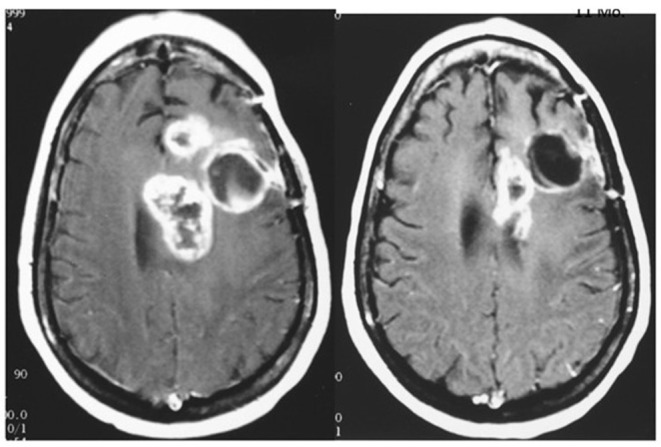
MRI of brain of a 42 year old female with recurrent glioblastoma showing significant mass reduction with free thyroxine depletion at 4 months. Left, pre-thyroxine depletion; right, 4 months later. The patient survived for 3 years. Reprinted with permission from Hercbergs et al. (4).
